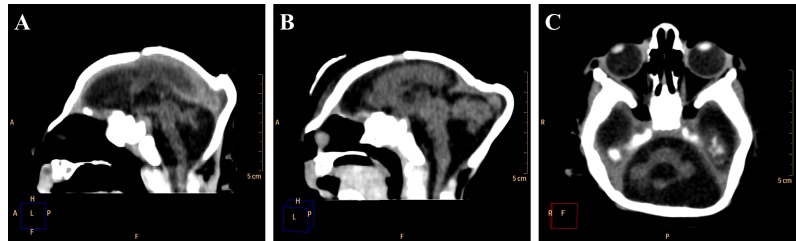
Figure 5. Sagittal (A and B) and axial (C) non-contrast brain CT showing cerebellar atrophy/hypoplasia.
